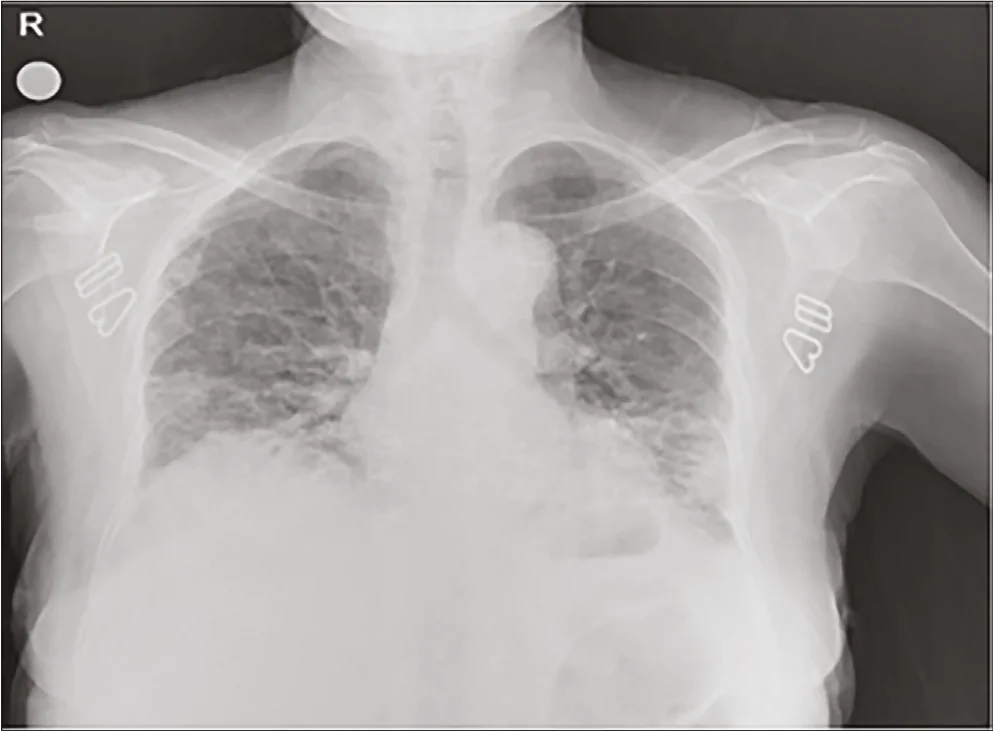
Chest X-ray before plasmapheresis.
